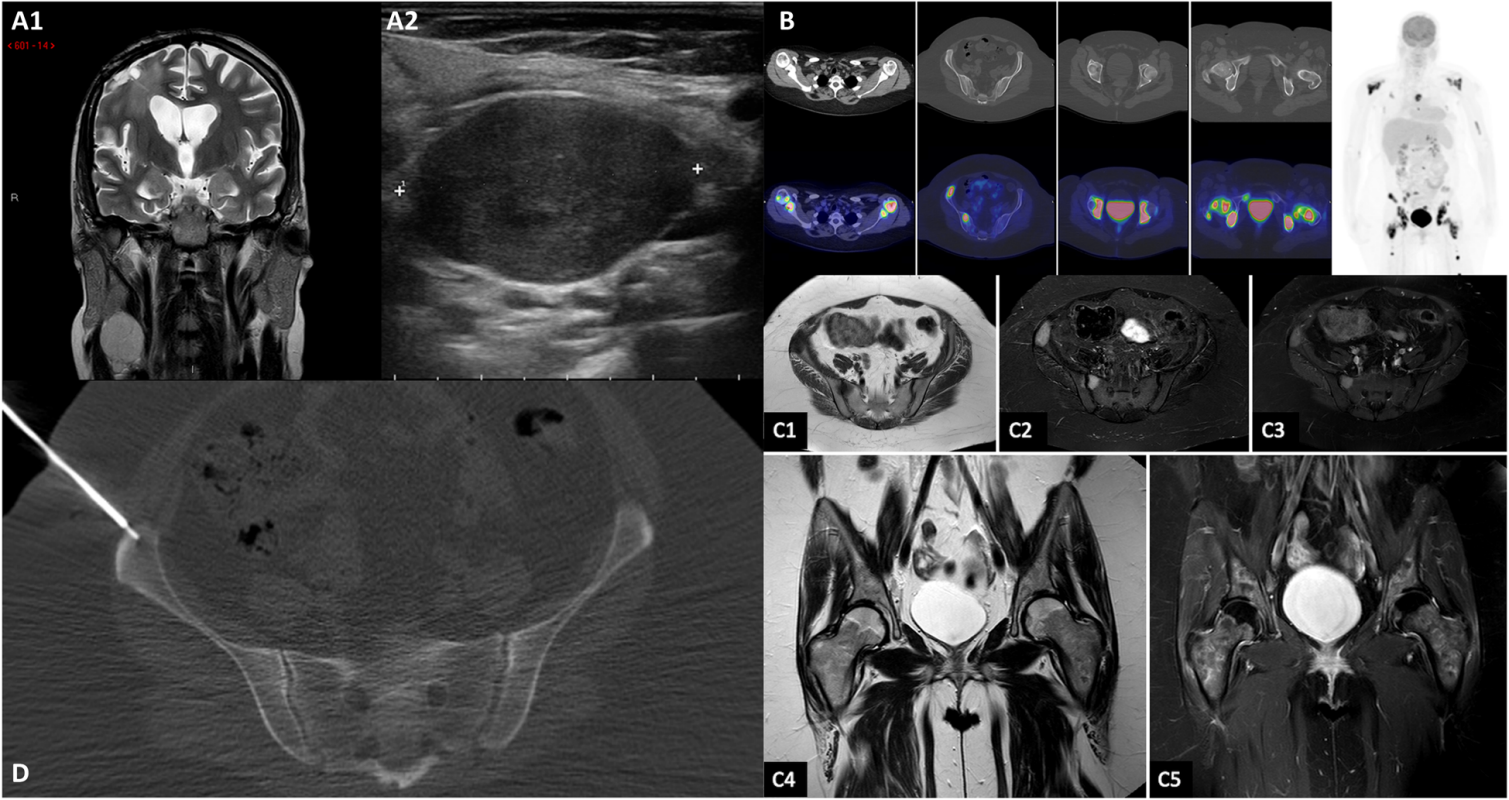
Brain and pelvic MRI, Ultrasound, Whole body 18F-FDG PET/CT scan, CT guided biopsy. Legend: Coronal T2-wighted image shows the contemporary stability of the right frontal lesion (A1) with the appearance of a right cervical mass (A1, red arrow). Ultrasound in the right lateral cervical site confirms that the lymph node is increased in size (maximum diameter of 3.5 cm) with pathological structure (A2). Whole body 18F-FDG PET/CT scan shows multiple increased 18F-FDG uptake areas (B). None of them correlates with significant osteostructural alteration on CT scan. Pelvic MRI demonstrates focal signal alterations at sacrum and iliac wing on the right side on T1-weighted axial (C1), STIR (C2), T1 SPIR with Gd (C3), and diffuse signal alteration of both hips and proximal third of the femurs on T2-weighted coronal (C4) and T1 SPIR with Gd (C5). CT guided biopsy performed at the right iliac wing, based on PET images and MRI (D)
